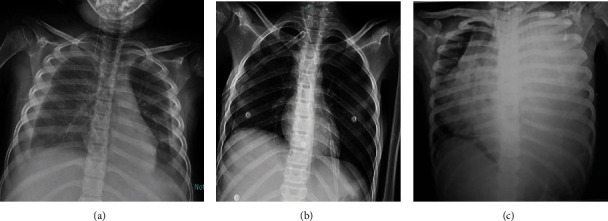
Chest X-rays of three patients with COVID-19 having pleural disease which is an atypical finding for pediatric COVID-19 infection that required intervention. (a) A 5-year-old female with a history of fever and cough. Chest X-ray shows bilateral pleural effusion. The patient was discharged after 12 days. (b) A 14-year-old female with associated tuberculous enteritis and tuberculous lymphadenitis was presented with fever and acute shortness of breath. Chest X-ray shows bilateral pneumothoraces; no parenchymal lung abnormality was detected on CT (not shown). The intercostal tube is also noted. The patient severely deteriorated and was transferred to the intensive care unit, was mechanically ventilated, and died. (c) A 5-year-old female was presented with fever, cough, and respiratory distress. Chest X-ray shows a homogenous opacity involving the left hemithorax associated with a marked mediastinal shift to the contralateral side in keeping with the left-sided pleural effusion. The patient underwent intercostal tube insertion, clinically improved, and was discharged after 15 days.
